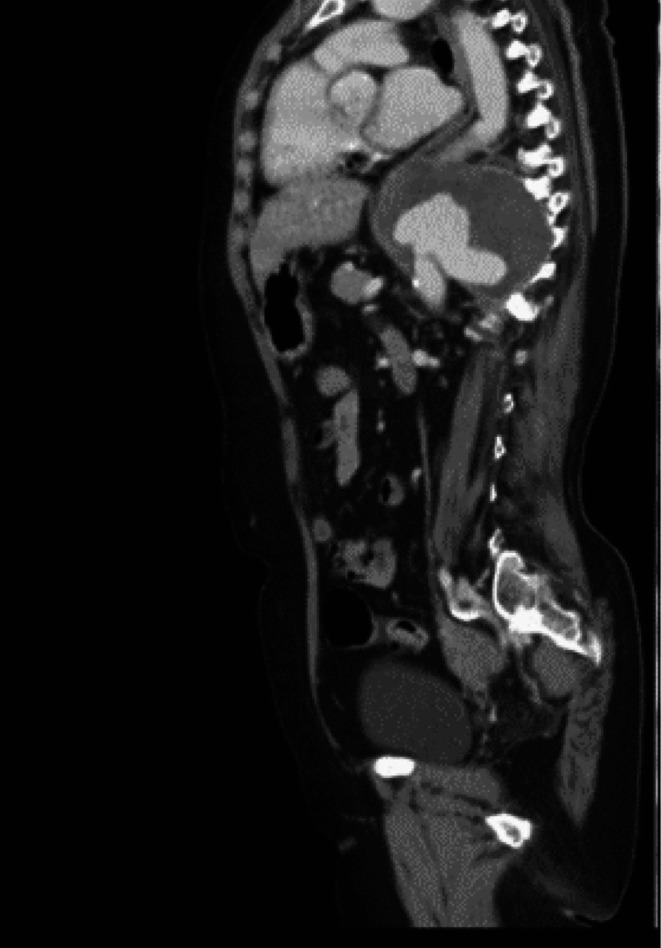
Preoperative sagittal CT reconstruction demonstrating near‐complete lytic erosion and collapse of the T9 to T11 vertebral bodies. This erosion results in a focal kyphotic deformity and anterior angulation of the spine.
